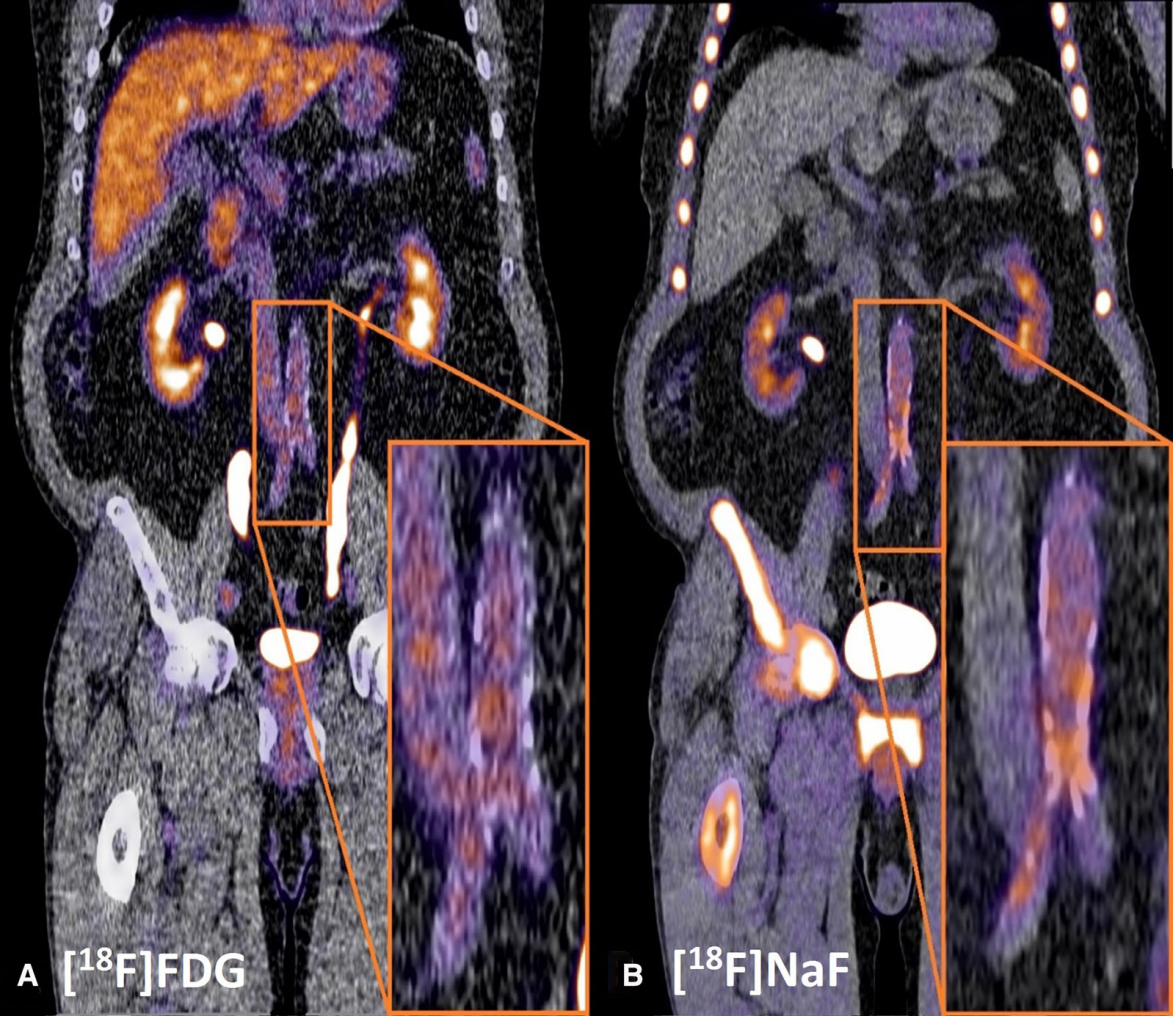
[^18^Fluor]fluordeoxyglucose ([^18^F]FDG) and five-year follow-up [^18^Fluor]SodiumFluoride ([^18^F]NaF) positron emission tomography/low dose computed tomography in a patient with type 2 diabetes mellitus. A Metabolic active cells are visualized by [^18^F]FDG uptake can be found in the liver, heart, and more focal in arterial tissue (pop-up). In arterial tissue, [^18^F]FDG uptake is a marker for inflammation which is considered an important factor in the development of CVD. B [^18^F]NaF uptake demonstrates skeletal activity and, next to that, this tracer also visualizes micro-calcification formation in the arterial wall (pop-up). Both nuclear tracers are excreted via the kidneys, ureters, and bladder. For image processing and analysis Affinity 2.0 was used (Hermes Medical Solutions)
